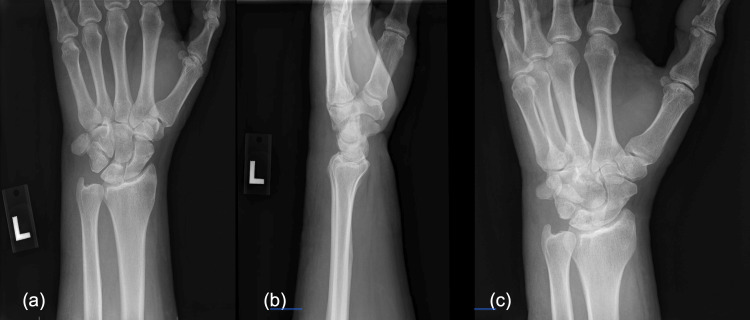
(a) AP, (b) lateral, and (c) oblique radiographs demonstrating dorsal ulnar dislocation of the pisiform without any associated fractures AP: Anteroposterior
